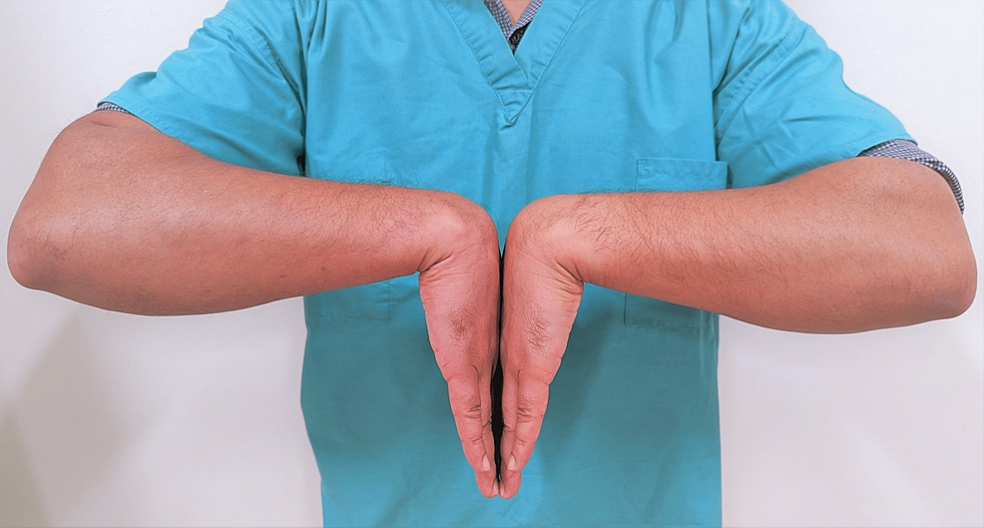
Phalen's test This is an original image from the ultrasound lab department of the American University of Antigua
